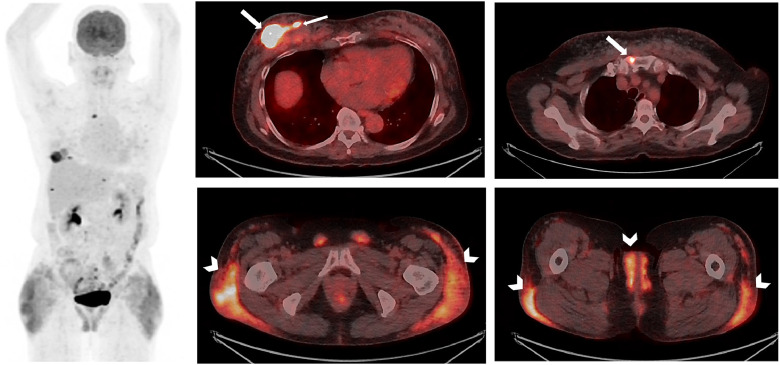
Following neoadjuvant chemotherapy, a progress FDG PET/CT was performed 6 months later. Intense metabolic activity in a new right medial breast lesion (SUVmax 8.2) (medial thin arrow) was demonstrated with interval partial reduction of metabolic activity in the remaining known 2 breast malignancies (SUVmax 15.0 to 10.1). There was reduced metabolic activity in the bilateral axillary, mediastinal, para-aortic, and left pelvic lymph nodes (SUVmax 6.7 to 2.1) as well as the sternal bony metastasis, favored to represent response to neoadjuvant chemotherapy (thicker arrows). Mild reduction in metabolic activity in the subcutaneous silicone injections (SUVmax 7.0 to 5.3), favoured variable silicone-related inflammation (arrowheads).
